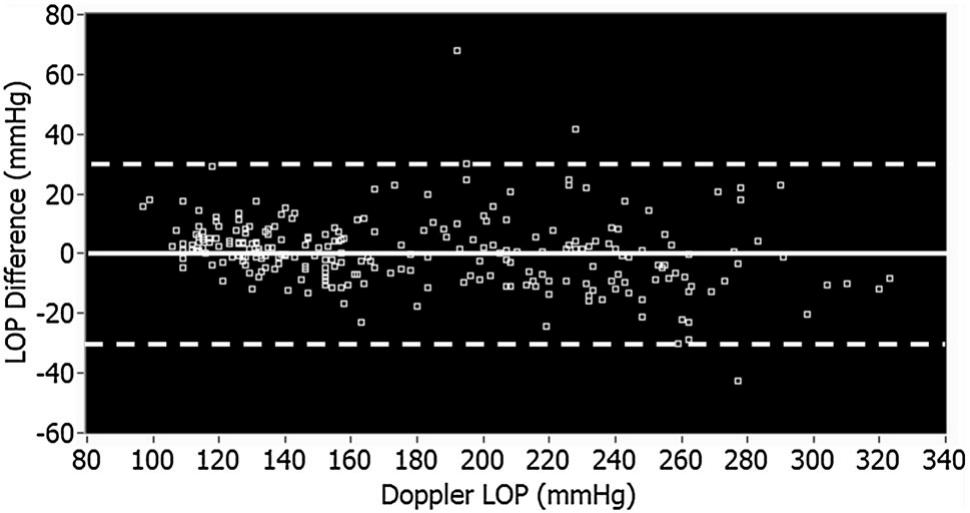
Bland–Altman Plot of LOP difference between proposed technique and manual Doppler technique for all limbs. Mean difference is shown (*solid line*) plus or minus two standard deviations (*dashed lines*)
